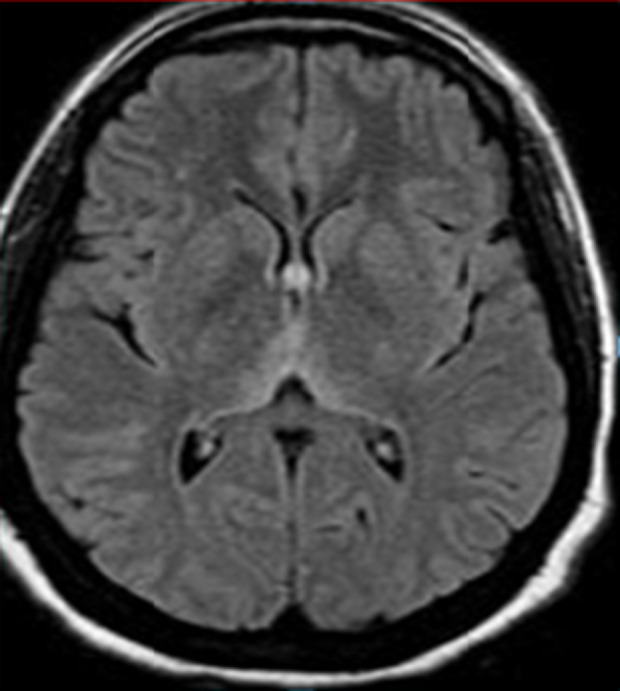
IRM encéphalique, en coupe axiale séquence FLAIR, aspect hyper intense de part et d’autre du 3^e^ ventricule
